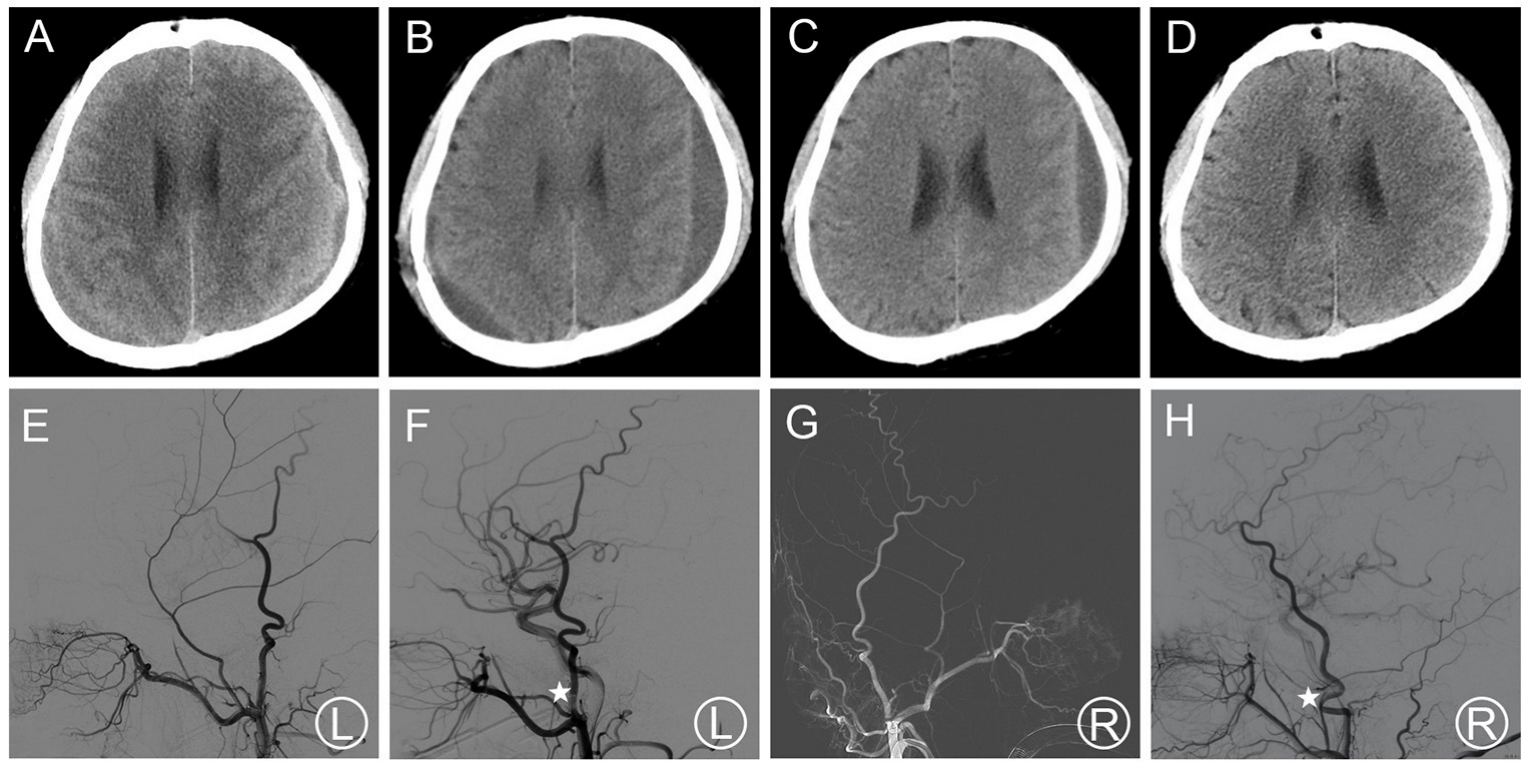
(A) Brain CT images of a hematoma in patient 2 before treatment. (B,C) Brain CT was repeated at 14 days after surgery and before the patient's discharge. (D) Follow-up brain CT was performed at 97 days. (E,F) Pre- and post-operative DSA presented left MMA and the left MMA disappeared (asterisk). (G,H) Pre- and post-operative DSA presented right MMA and the right MMA disappeared (asterisk). DSA, digital subtraction angiography; CT, computed tomography; MMA, middle meningeal artery.
